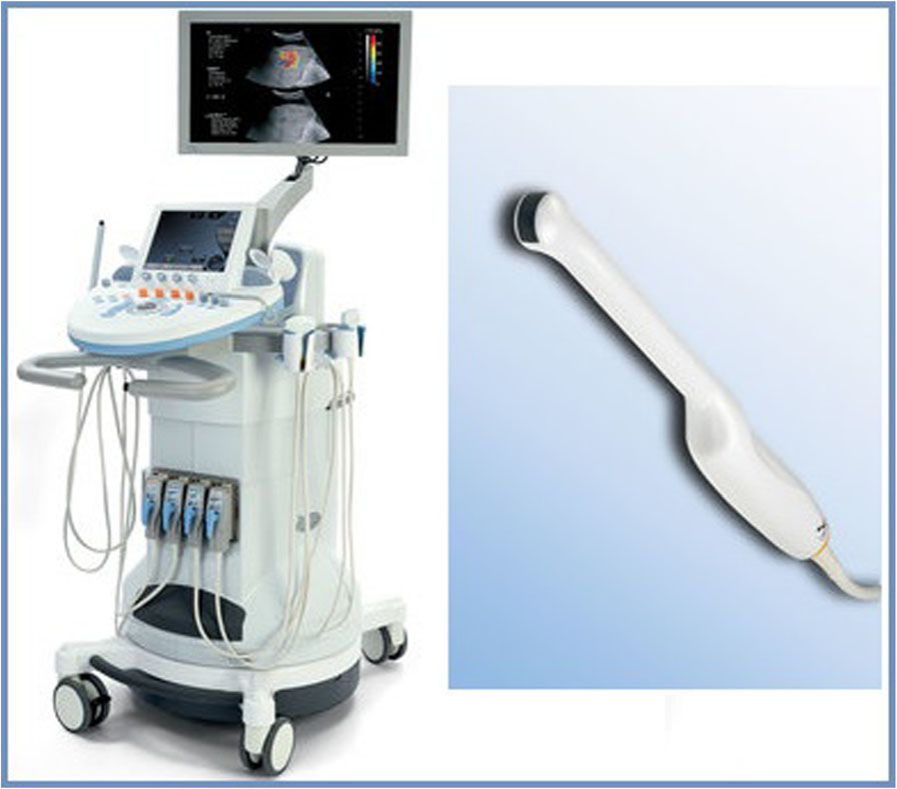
The ultrasound system and endorectal probe. Legend: Ultrasound scanner (Aixplorer®, Supersonic Imagine, Aix-en-Provence, France) and endorectal probe (SuperEndocavity™ SE12–3 with number of elements: 192 and bandwith: 3–12 MHz, Supersonic Imagine, Aix-en-Provence, France) used for the purpose of this study
